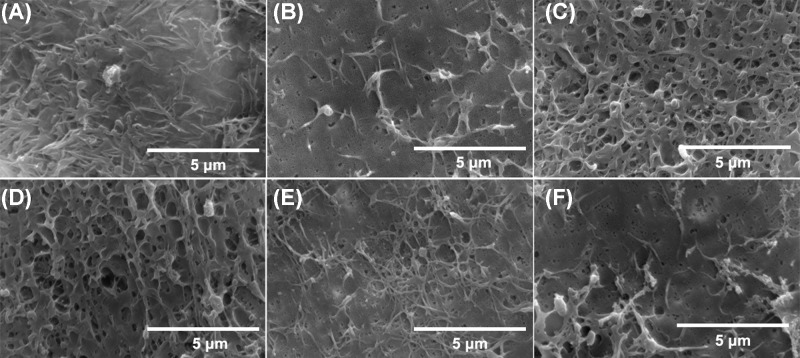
Cell membrane morphology analysis MDA-MB-231 cells untreated (A), cells 0 min after ultrasound treatment (B), and 0 min (C), 5 min (D), 15 min (E) and 30 min (F) after UTMD treatment were fixed with 3% glutaraldehyde and subjected to SEM analysis.
